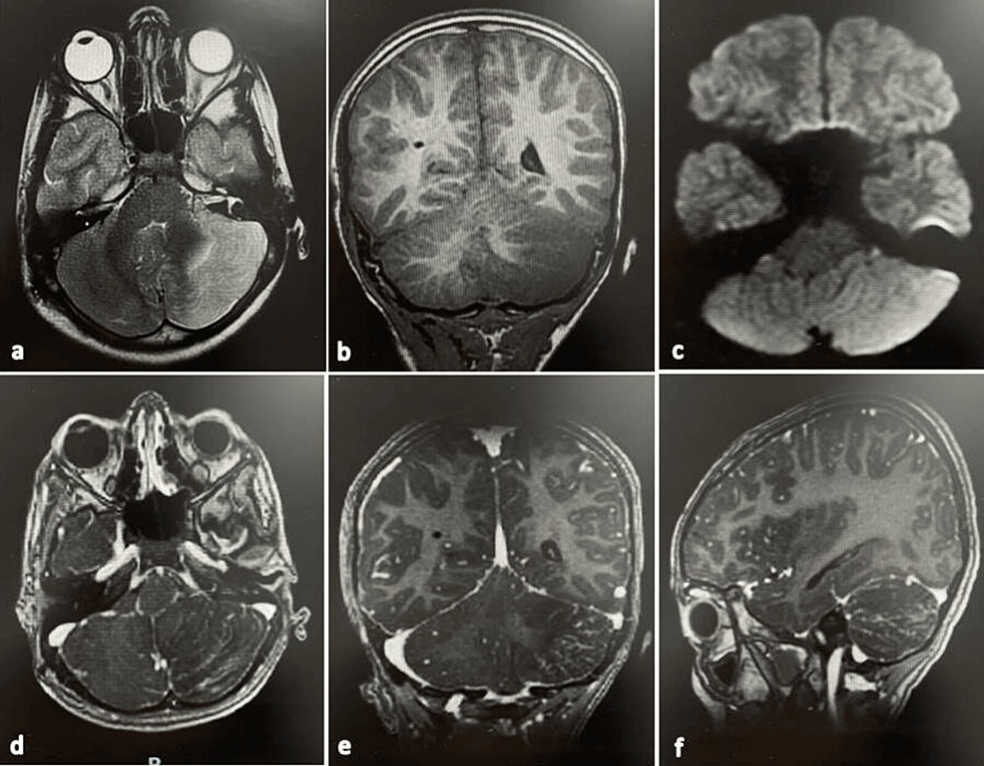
Pre-operative brain MRI of an eight-year-old boy with Lhermitte-Duclos disease (LDD) (a) Axial T2-weighted image showing patchy high-signal lesion in the left cerebellum with mass effect and compression of the fourth ventricle. The classic tiger-stripe appearance is considered typical for LDD; (b) Coronal T1-weighted image showing low-signal lesion in the left cerebellum (typical finding); (c) Axial diffusion weighted imaging (DWI) showing high signal (restricted diffusion); (d–f) Axial, coronal, and sagittal contrasted T1-weighted images.
